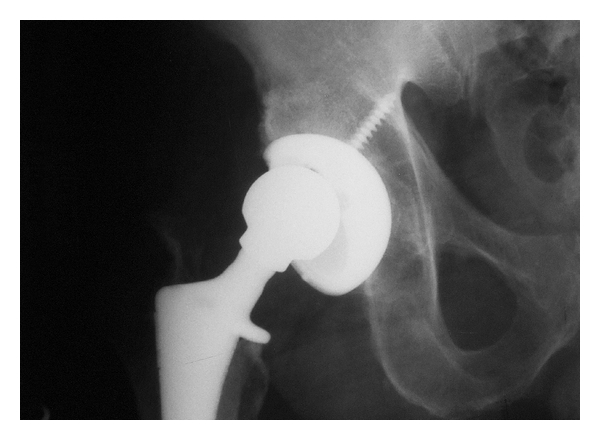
Patient 2: anteroposterior radiographs demonstrating eccentric placement of the femoral head within the acetabular polyethylene and excessive acetabular component abduction.
